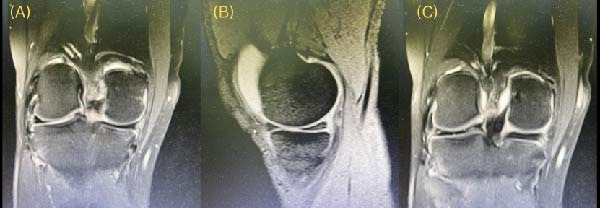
Follow‐up MRI of the right knee (Case 1) obtained 6 weeks postoperatively. (A, C) Coronal proton‐density fat‐saturated and (B) sagittal T2‐weighted images demonstrate intact healing of the previously repaired medial meniscus and a new nonarticular tear of the posterior horn of the lateral meniscus, with no loose bodies or suture migration identified.
